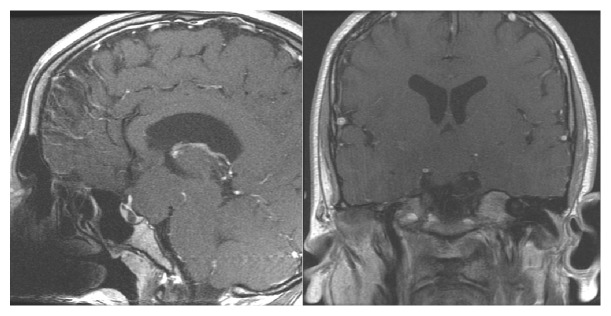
Sagittal and Axial MRI with contrast showing isointense, nonenhancing hypothalamic mass.
